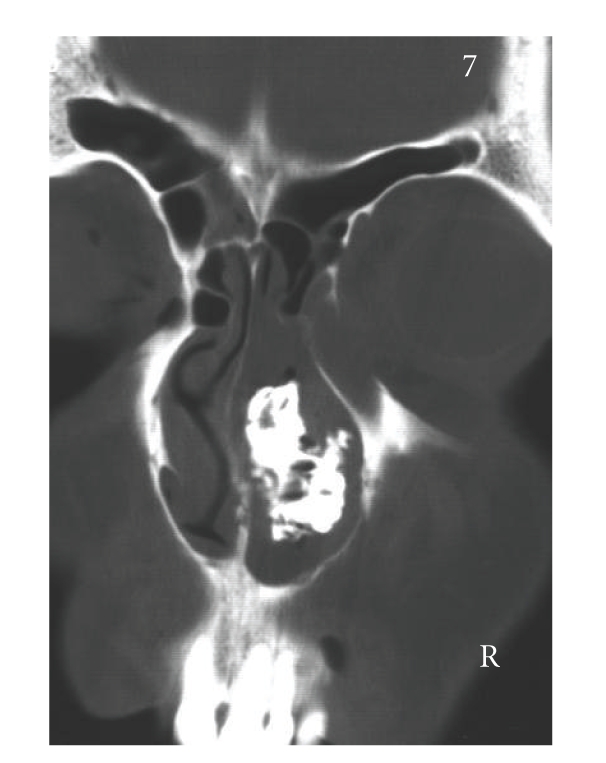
Coronal CT showing a calcified space-consuming lesion occupying much of the right nasal cavity.
